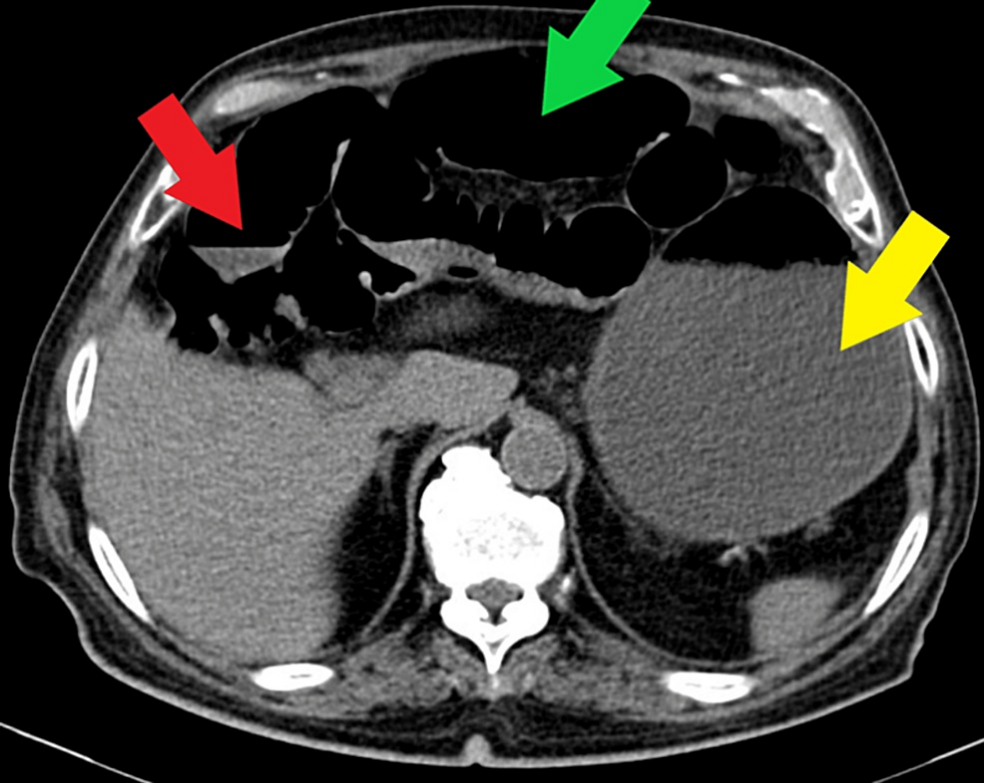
Non-contrast CT scan of the abdomen showing air fluid levels, distended small bowels, and an enlarged stomach suggesting an obstruction. Red arrow: air-fluid levels; Green arrow: distended small bowels; Yellow arrow: enlarged stomach
